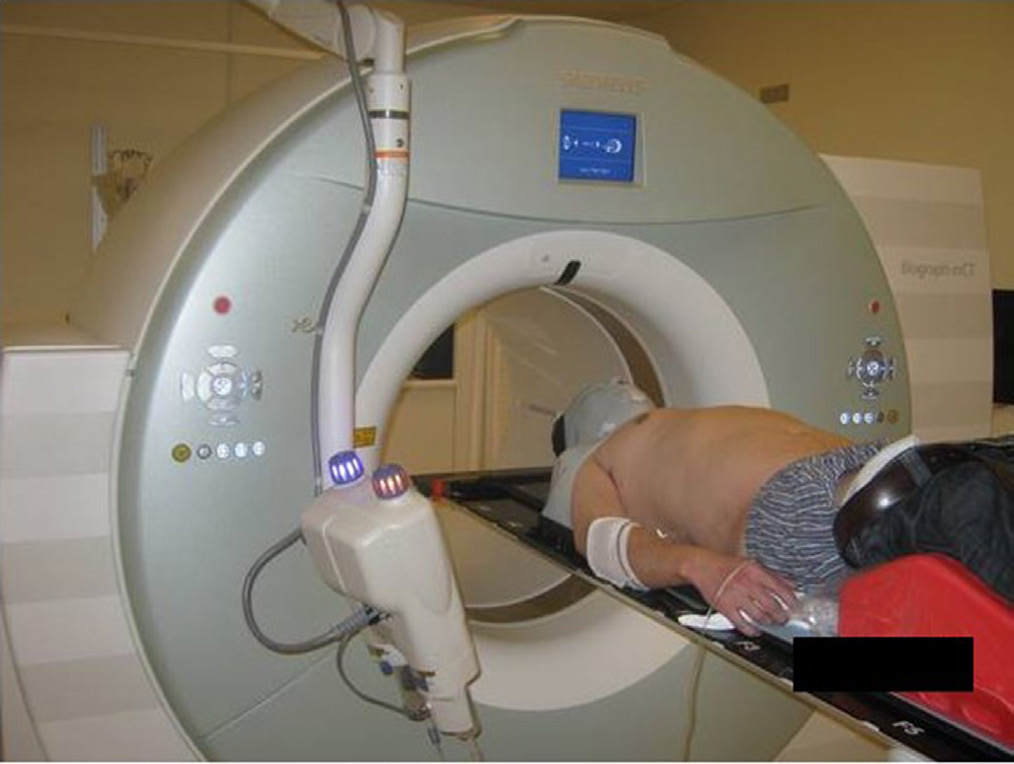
Example of pre-chemotherapy image acquisition satisfying INRT conditions. The PET/CT scanner is fitted with a flat table top. The patient position mimics that of the anticipated treatment position for radiotherapy. Here, the patient is scanned with arms down and a thermoplastic mask covering the head and shoulders. A knee pad (red) is used to increase comfort. This approach necessitates a close collaboration between the nuclear medicine and the radiotherapy teams (for example, radiotherapy radiographers may be present during pre-chemotherapy PET/CT alongside nuclear medicine technologists).
